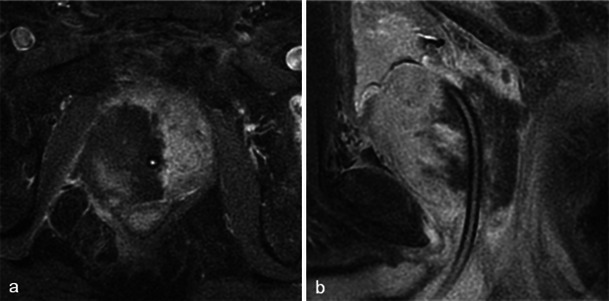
Urethral necrosis. Right photodynamic therapy in a 60-year-old patient with a PSA level of 12.9 ng/ml and Gleason 6 adenocarcinoma (positive base biopsies, right middle prostate). Day-7 post-treatment axial (a) and sagittal (b) T1 FS contrast-enhanced MR images do not show the normal periurethral ring of enhancement; the patient developed acute urinary retention treated by urinary catheter
